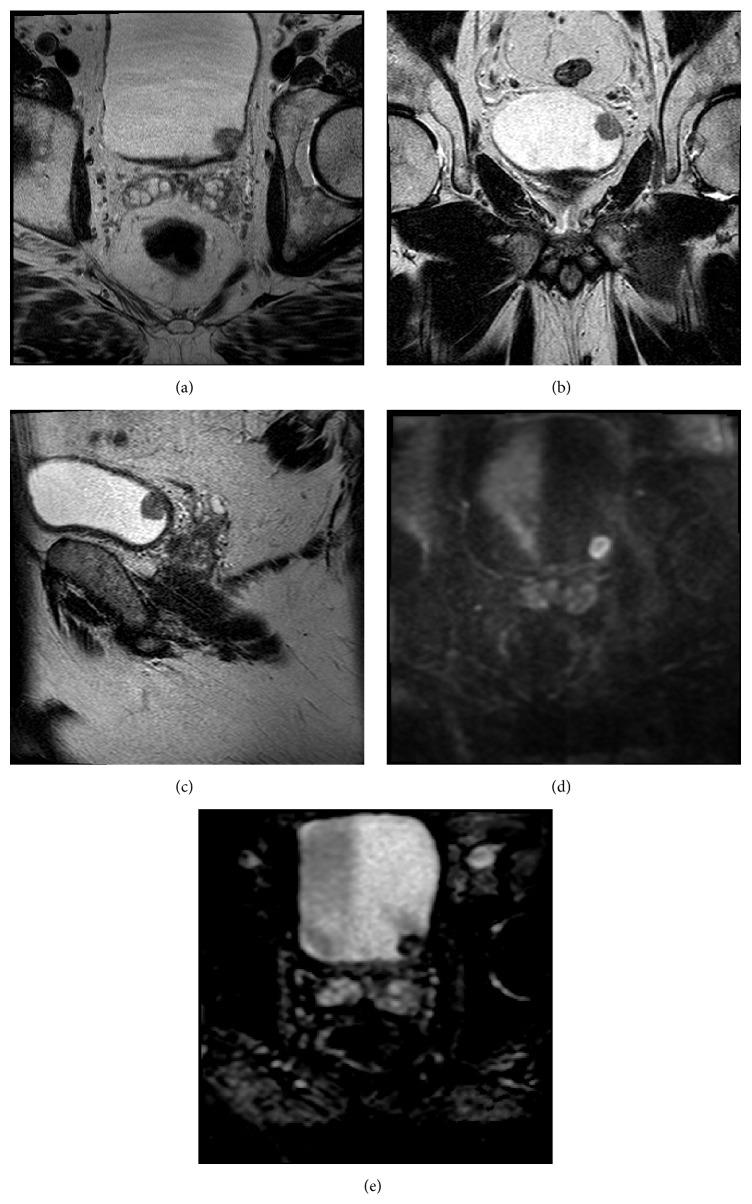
((a), (b), and (c)) Axial, coronal, and sagittal T2 weighted images showing a nodule in left bladder base as a papillary mass of the bladder wall. ((d) and (e)) DWI B2000 and ADC images showing restricted diffusion of bladder mass.
